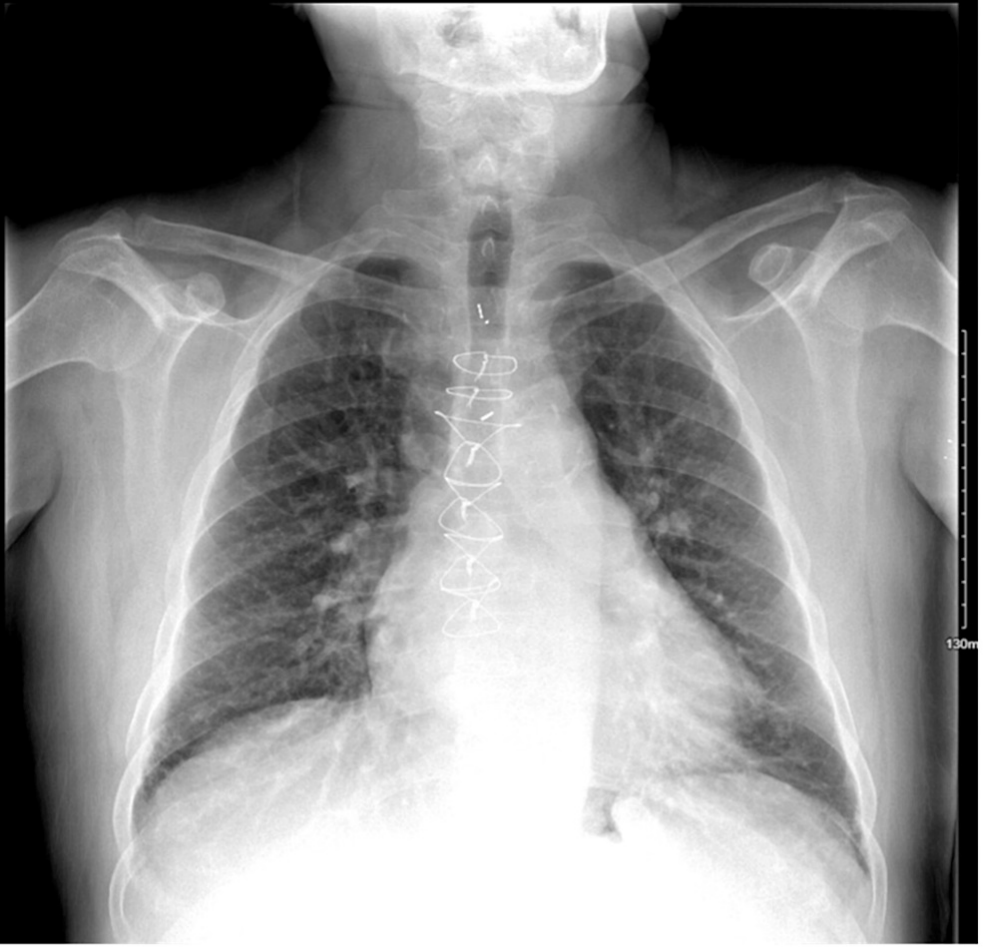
Chest X-ray findings. Chest X-ray without any imaging evidence of obvious lung or cardiac pathology.
